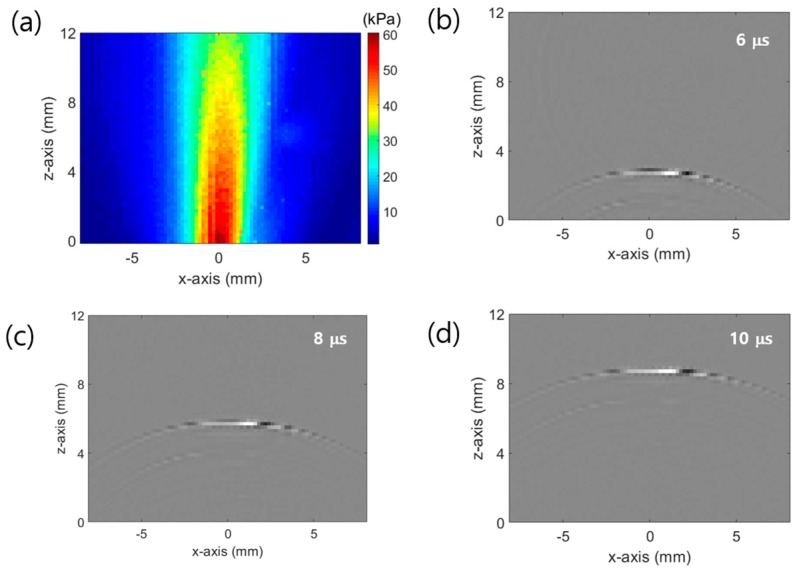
(a) Measured optical ultrasound pressure field generated by the CNTs in water medium; Sound wave propagation images at (b) 6, (c) 8, and (d) 10 μs.
